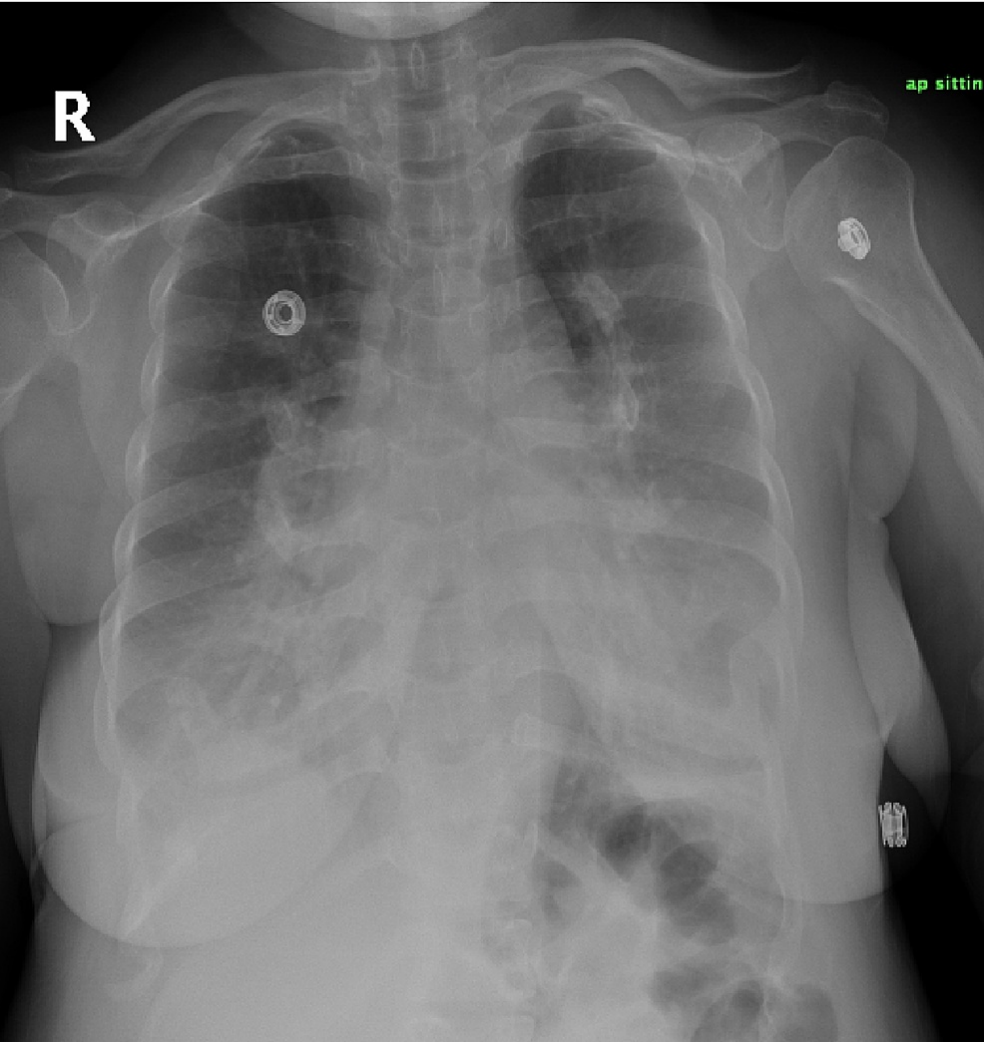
Enlarged cardiac shadow with hazy heart boundaries, bilaterally obliterated cardiophrenic and costophrenic angles, lower zones opacified in comparison to upper zones are seen. These findings are suggestive of alveolar airspace disease with bilateral pleural effusion, consistent with diagnosis of congestive heart failure. However, the CT study revealed something different. CT, computed tomography
